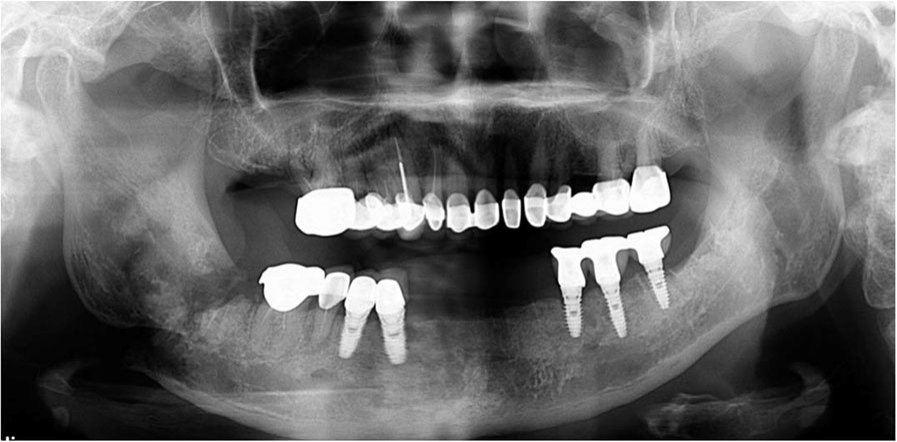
Panoramic radiograph showing pathologic fracture occurred at the right mandibular angle area
